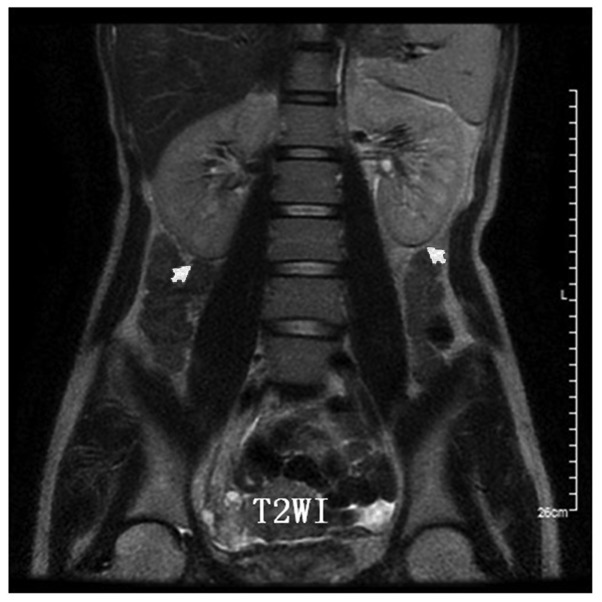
Abdominopelvic magnetic resonance imaging scan showing normal bilateral kidneys (white arrows indicating the normal kidneys).
